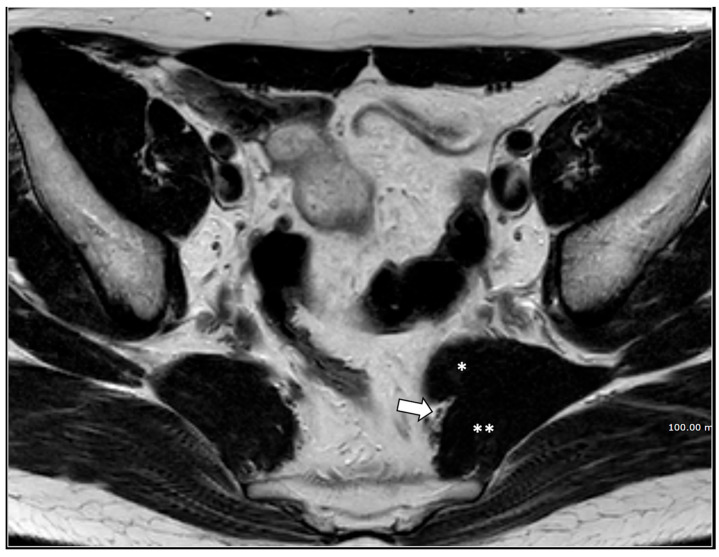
A 37-year-old male with pudendal neuralgia. Axial T2-weighted scan through mid-pelvis shows hypertrophy of the left piriformis muscle. Roots of the lumbosacral plexus lie on the anterior surface of the muscle. On the other hand, S2 and S3 roots of the pudendal plexus (arrow) can be seen along the medial surface of the muscle within an incomplete canal created by the two bellies (* and ) of the muscle.
